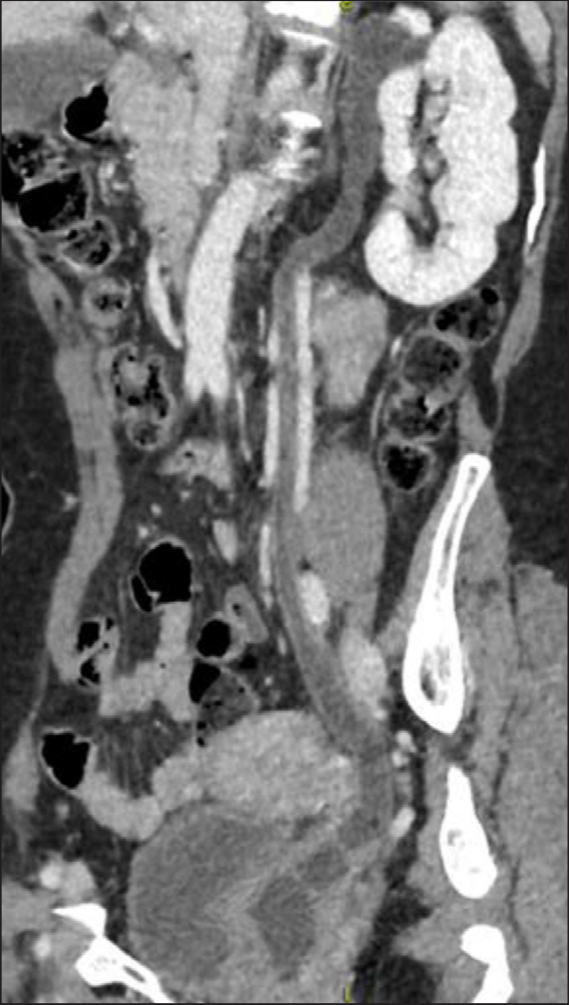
Curved reformatted image of the contrast-enhanced computed tomography shows the full-length ectopic dilated ureter and the left side with proximal implantation on the left upper renal pole and distal implantation on the proximal third of the vagina.
